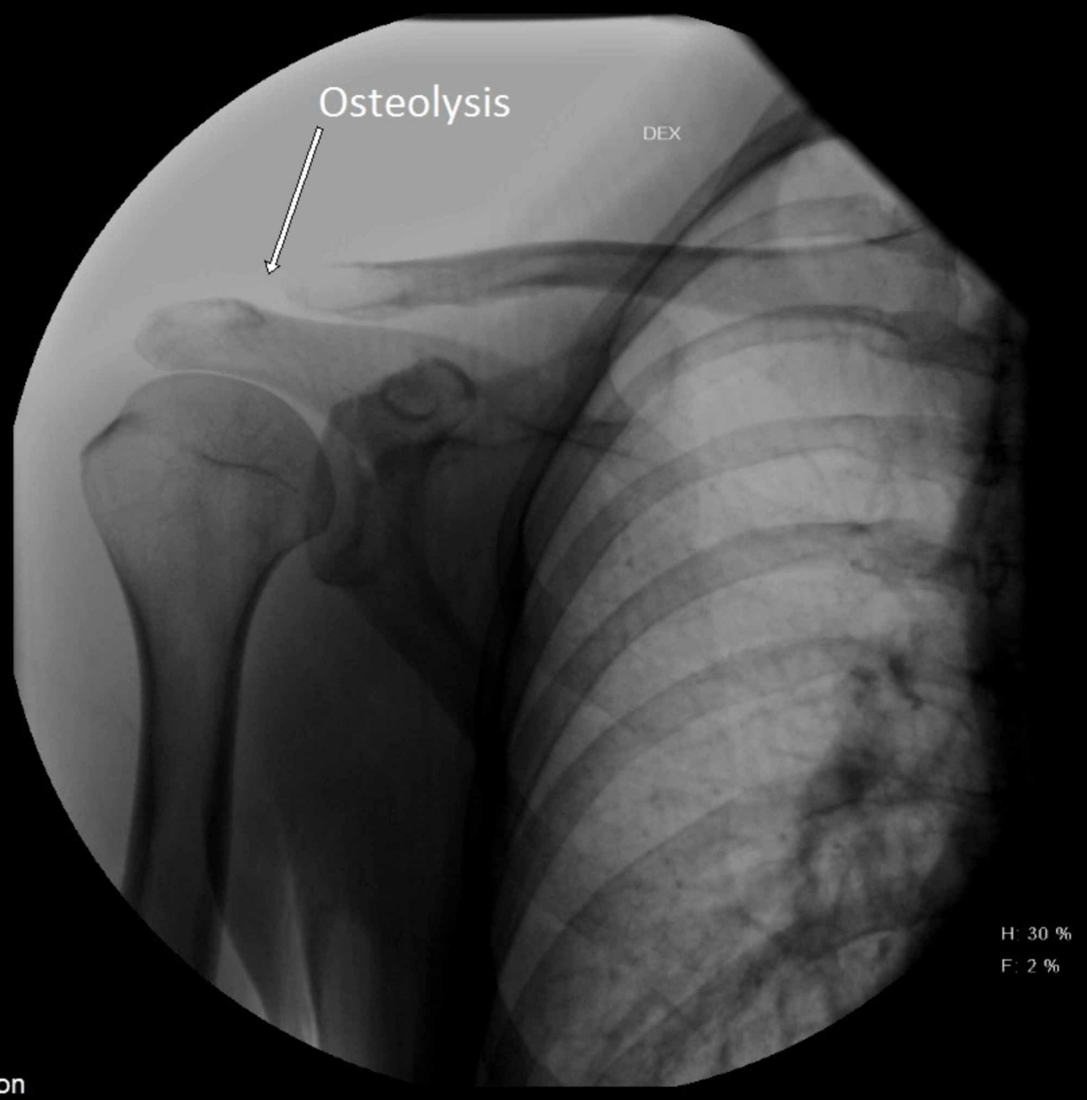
A radiograph of a 31-year-old male patient 16 months after ACJ reconstruction with suture loop. Osteolysis of the acromial end of the clavicle is present. ACJ, acromioclavicular joint
